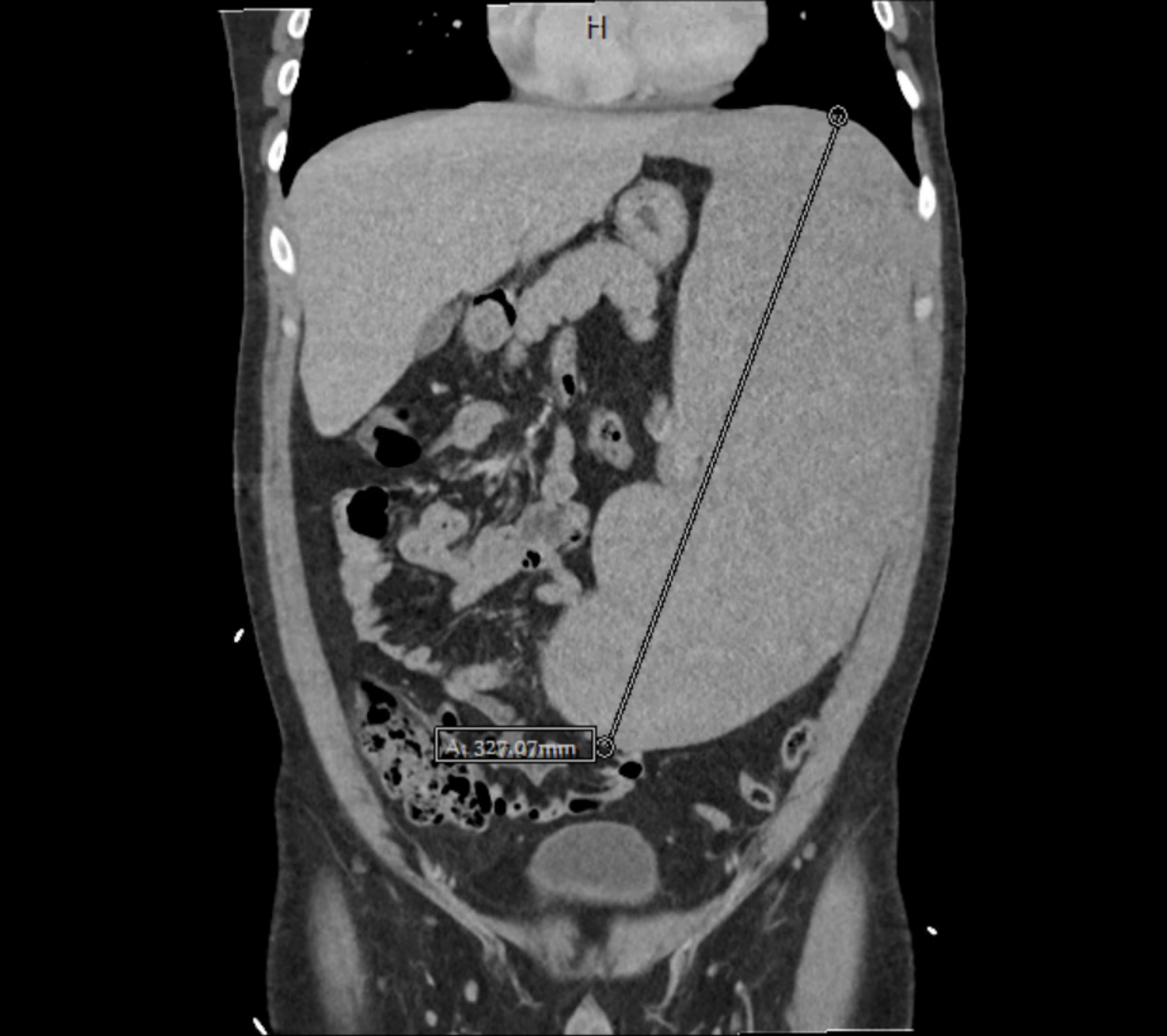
CT abdomen and pelvis with IV contrast demonstrating splenomegaly with largest diameter of 32.7 cm in the craniocaudal direction.
